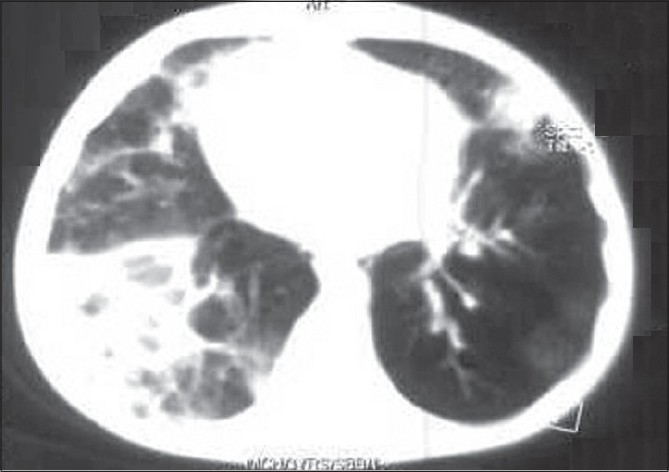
CT chest at the end of 3 weeks of illness showing multiple areas of consolidation in both the lung fields with ground glass opacities and mediastinal lymphadenopathy.
